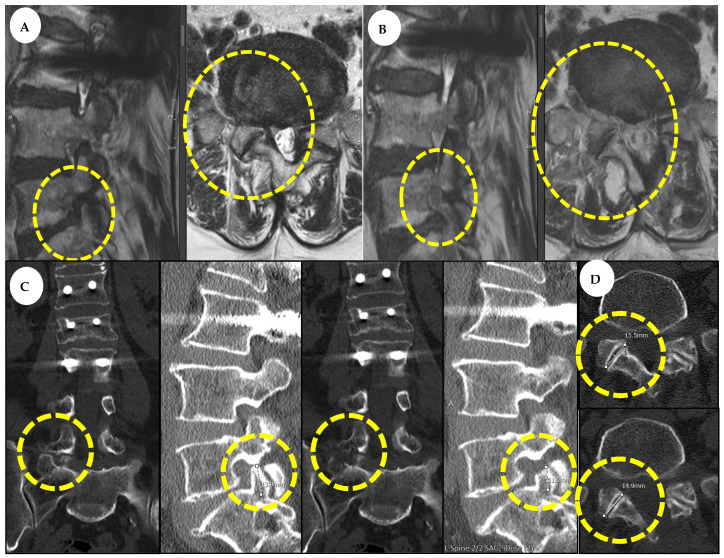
Radiological difference after two-way BESS decompression. The yellow circles indicate the lesion areas where preoperative and postoperative changes can be observed. (A) Pre-operative MRI; right-side L5-S1 lateral recess and extraforaminal stenosis were observed. (B) Post-operative MRI; sufficient decompression in the right side of L5-S1 was observed. (C) Pre-operative and post-operative coronal and sagittal cuts from CT scan; SAP tip was decreased by only 0.6 mm. (D) Pre-operative and post-operative axial cut from CT scan; joint length was decreased by only 2.9 mm. MRI, magnetic resonance imaging; CT, computed tomography; SAP, superior articular process.
